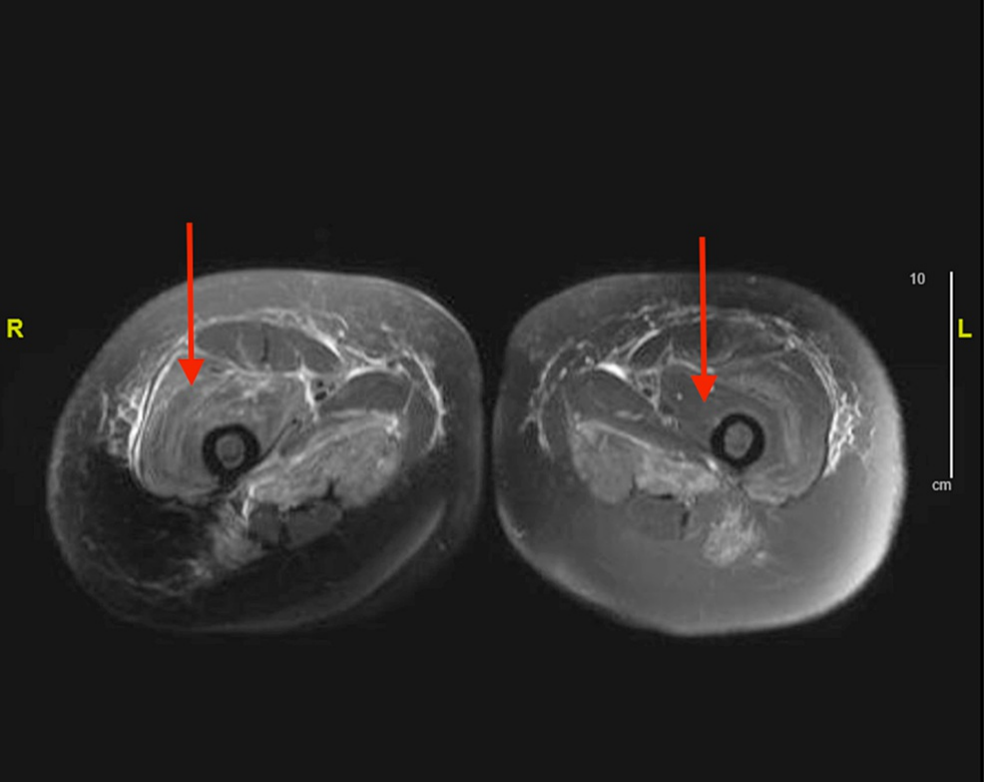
Axial MRI of pelvis Arrows demonstrate muscular edema of the thighs bilaterally.
